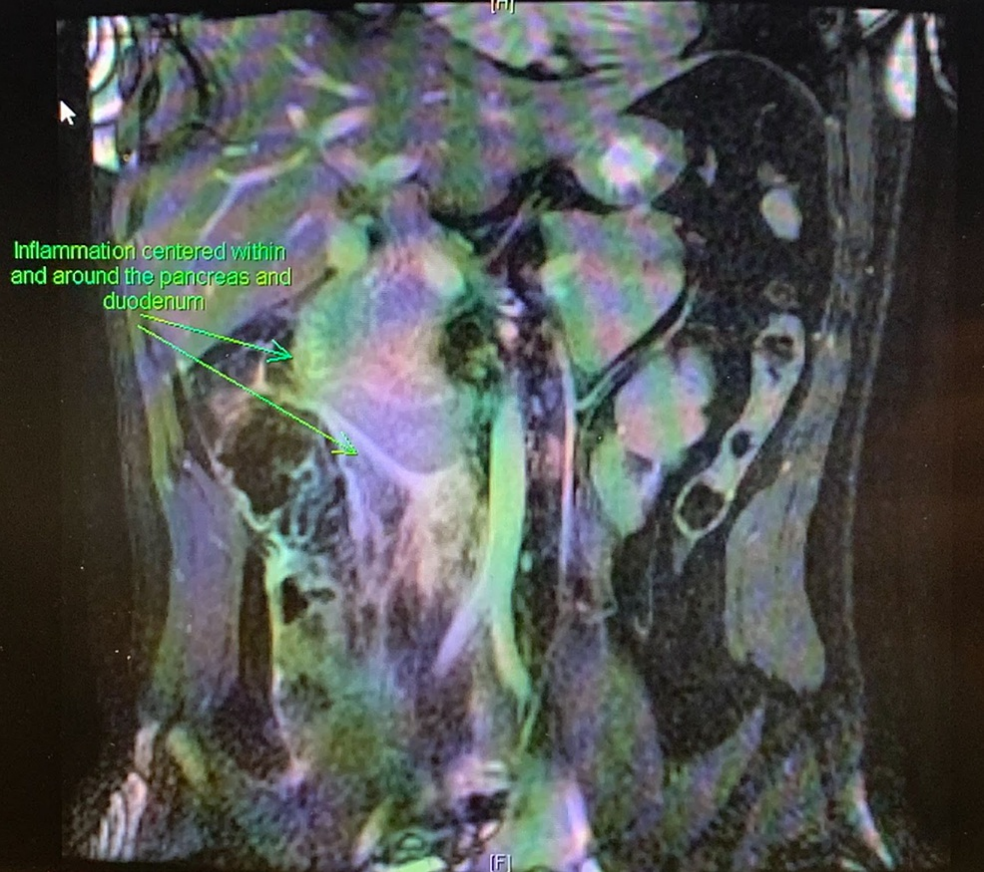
T2-weighted MRCP of the abdomen with fat saturation Coronal view magnetic resonance cholangiopancreatography (MRCP) of the abdomen highlighting inflammation centered within and around the pancreas and duodenum.
